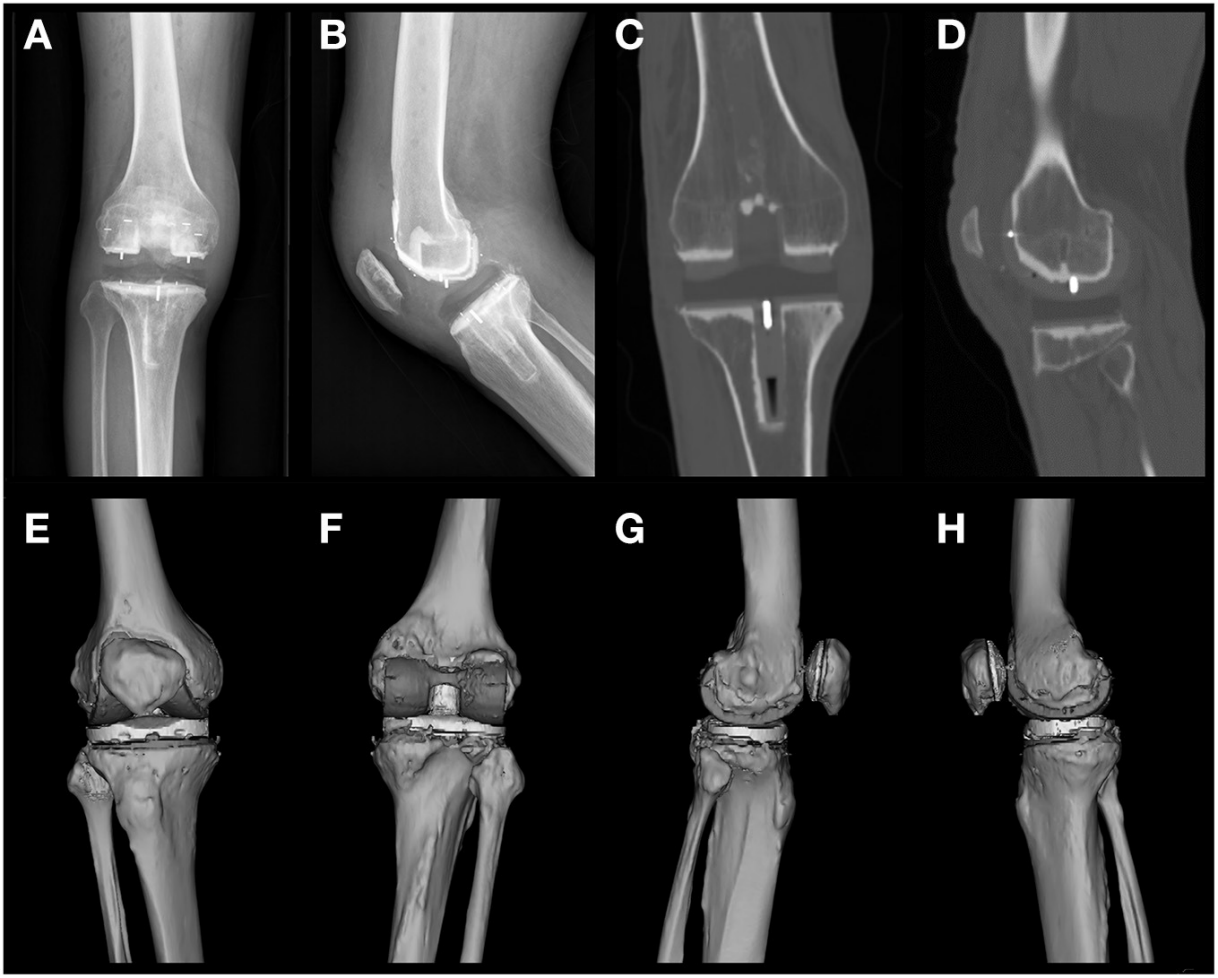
Postoperative X-rays of frontal and lateral views of patient 1 (A,B), images of postoperative CT coronal intermediate and lateral condyle central layers (C,D), and a preview of the 3D reconstructed model (E–H) are shown. CT level selection: coronal, sagittal, and axial positions consistent with the joint force lines. The most central metal line was selected according to the position of the central tibial plateau prosthesis and the central lateral femoral condyle. The most central position of the metal wire was selected as the intercept plane (C). Mimics reconstruction software was used to arrange the viewing angles in the order of anterior, posterior, lateral, and medial positions (E–H).
